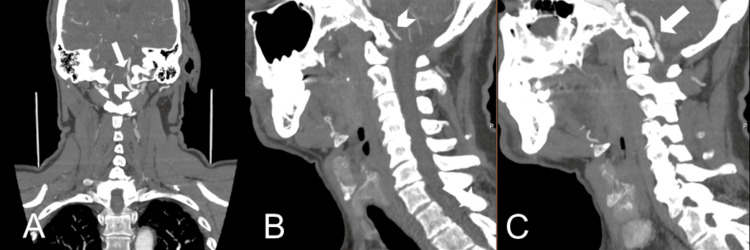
CTA of the head and neck on admission. A: coronal image; B: sagittal image from the right side; C: sagittal image from the left side. A hypoplastic right vertebral artery is seen in panels A and B (arrowheads). High-grade stenosis involving the distal left vertebral artery (intracranial V4 segment) is visualized in panels A and B (arrows).
